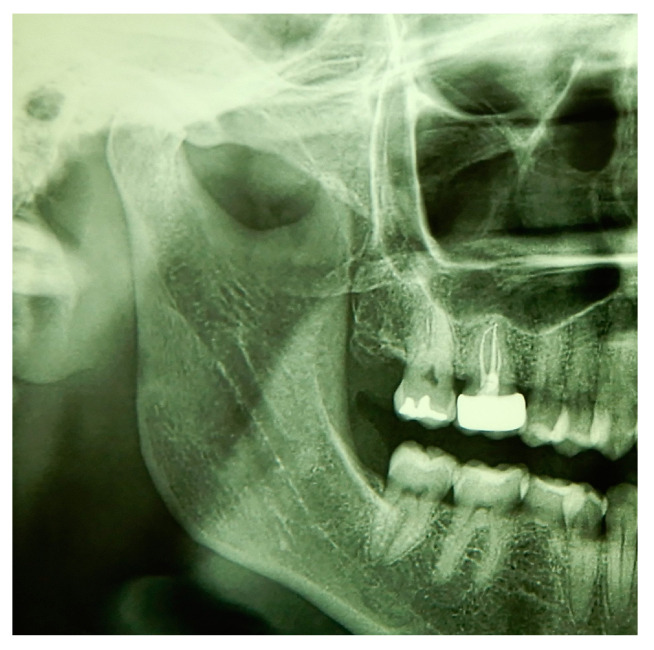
Radiographic control with orthopanoramic X-ray 5 years after auto-transplantation of the third molar.
